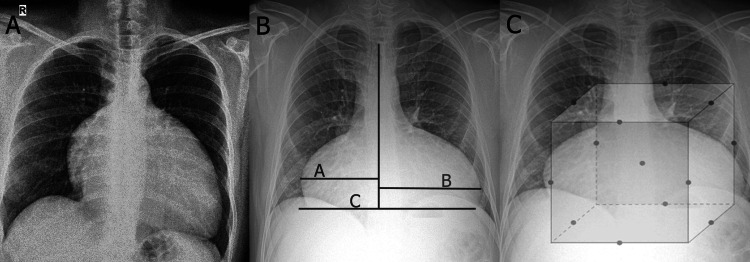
Chest radiographic findings. (A) Globe-shaped cardiomegaly with indistinct great-vessel borders. (B) Increased cardiothoracic ratio. (C) Box-shaped cardiac silhouette with right atrial enlargement, an appearance associated with a poorer prognosis when the cardiothoracic ratio exceeds 0.65.
